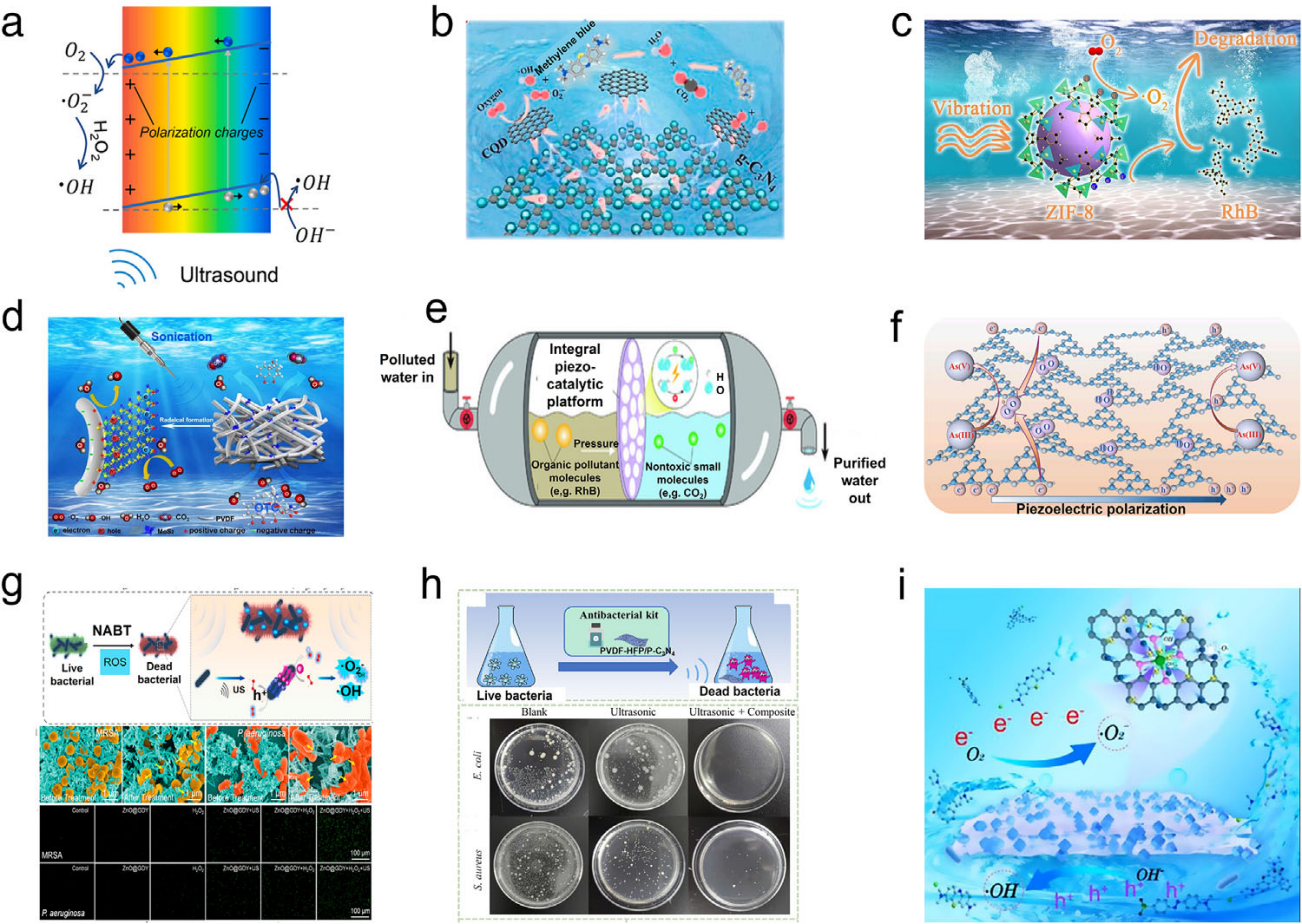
a) ROS generation by g‐C_3_N_4_ under ultrasound. Reproduced with permission.^[^
